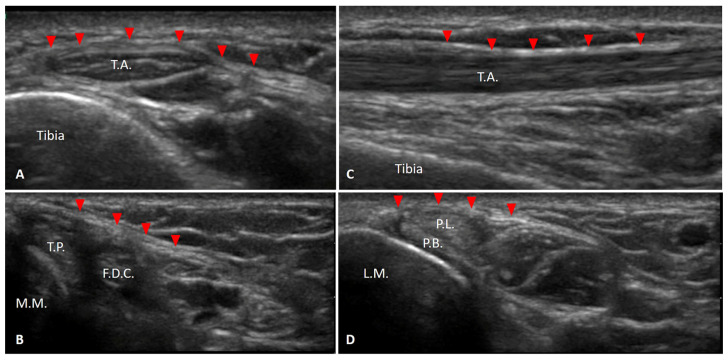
Ultrasound imaging of ankle retinacula. (A) Superior extensor retinaculum (short axis). (B) Flexor retinaculum. (C) Superior extensor retinaculum (long axis). (D) Superior peroneal retinaculum. T.A.: tibialis anterior tendon; T.P.: tibialis posterior tendon; F.D.C.: flexor digitorum comunis tendon; M.M.: medial malleolus; L.M.: lateral malleolus; Red triangles = retinaculum.
